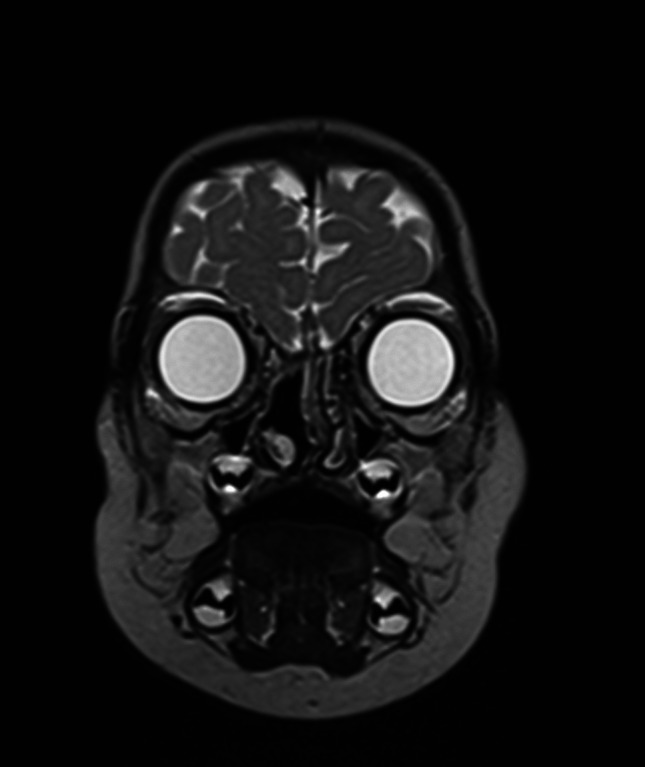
T2 weighted MRI scan in coronal plane ten months after surgery
